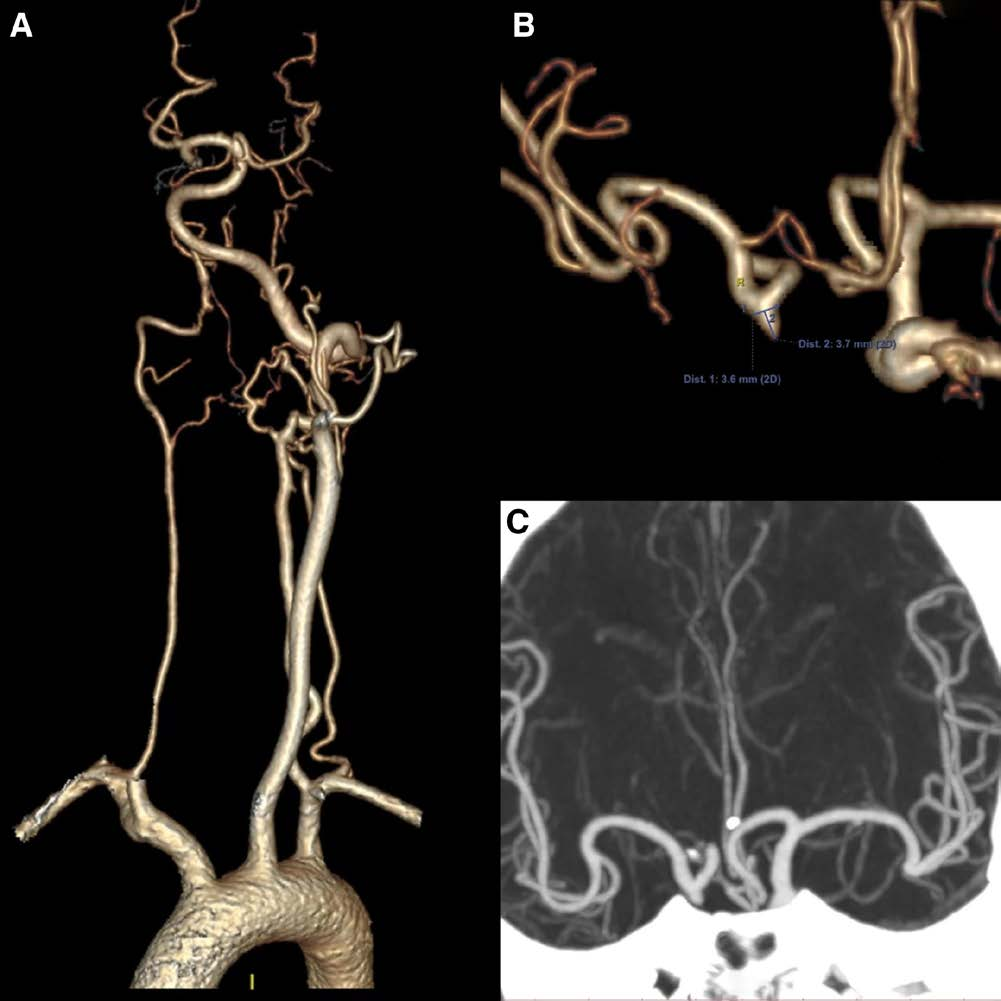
CT angiography showing that the right vertebral artery was slightly small, and the left vertebral artery was dominant. Main trunks disappeared at the foramen magnum and formed microvascular networks supplying blood to the skull (A). The left deep carotid artery formed a microvascular network at the foramen magnum and merged into the left vertebral artery (A).The vertebra-basal artery was dilated and tortuous (B). An aneurysm formed in the posterior traffic segment of the right internal carotid artery (C). CT angiography = computed tomography angiography.
